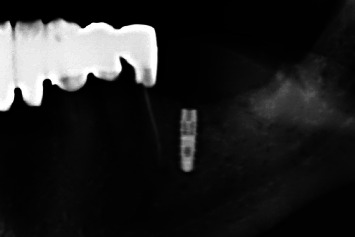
Regio #19-postoperative X-ray control (detail from panoramic tomogram).
